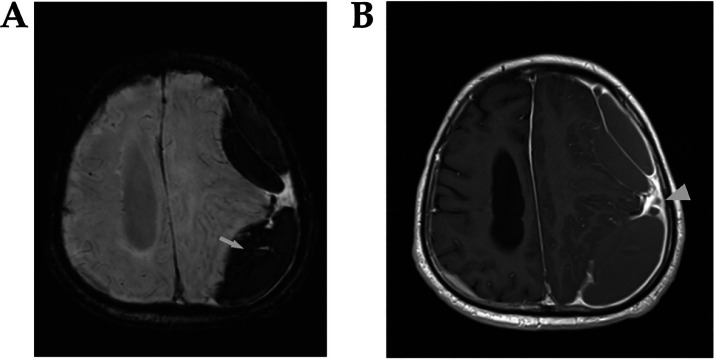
(A) The brain MRI susceptibility weighted imaging sequence axial section reveals a linear blooming signal, indicated by the “arrow,” which suggests hemorrhagic content. (B) The T1-weighted axial section with IV contrast displays dura enhancement, marked by the “arrowhead,” surrounding both the subdural collection and the cystic lesion.
